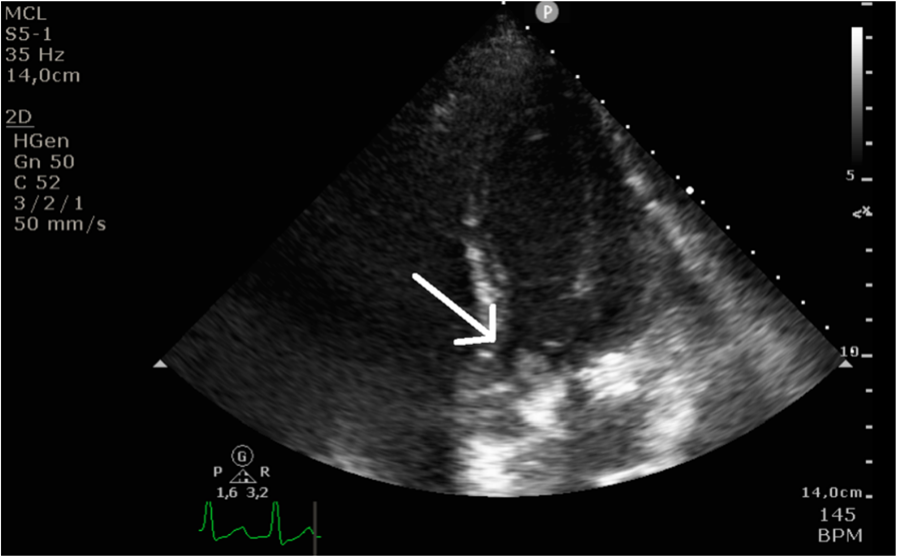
Transthoracic echocardiography showing a mobile structure adhered to the native aortic valve (white arrow)
